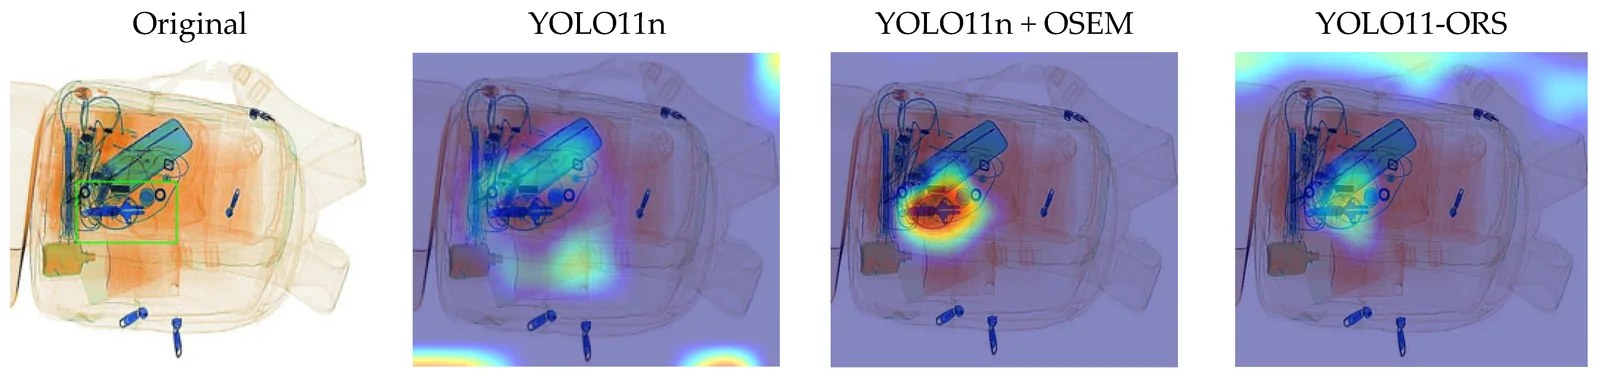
Class-specific Grad-CAM visualization for an occluded prohibited item on the OPIXray dataset. From left to right: original X-ray image, YOLO11n, YOLO11n + OSEM, and YOLO11-ORS. The green bounding box indicates the ground-truth target used to determine the visualization category.
